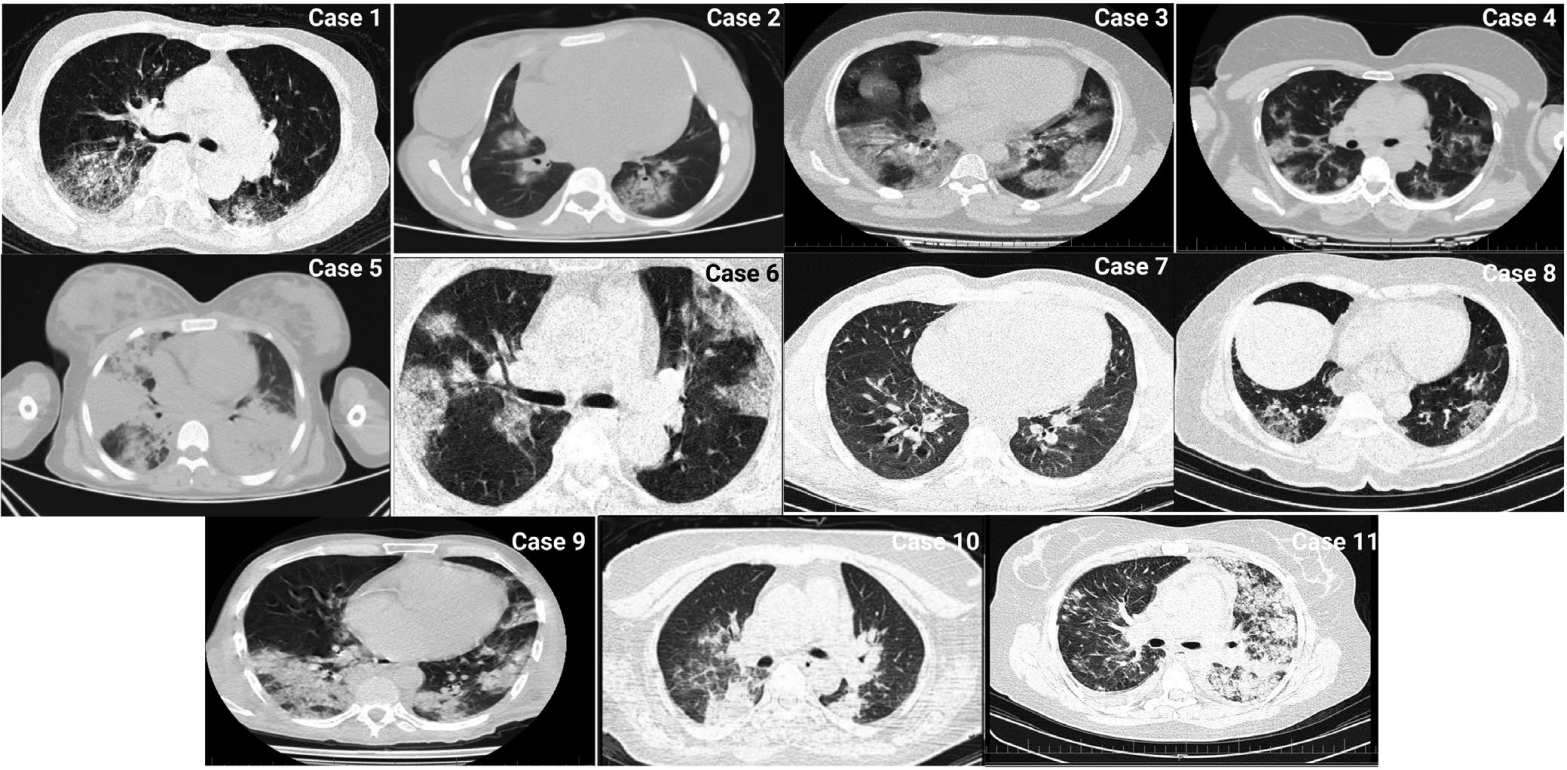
Chest CT scans show diffuse bilateral ground‐glass opacities (GGO) and basal consolidations in lung field
